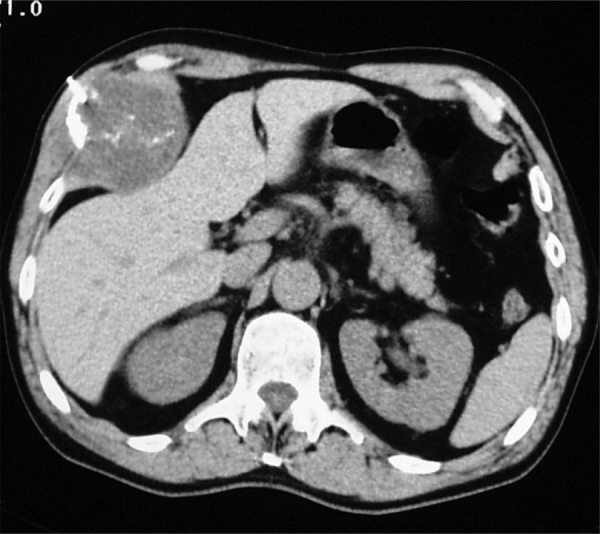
A CT-guided biopsy of a mass with cystic areas and calcifications originating from the 8th rib revealed a malignant chondral tumor.
